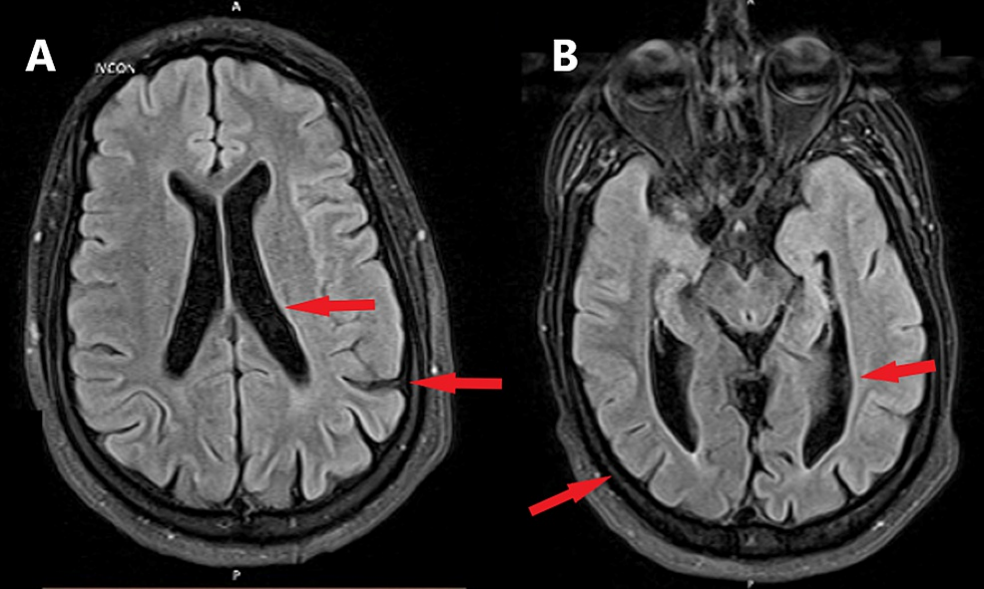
Axial brain MRI showing ventricular enlargement and diffuse cerebral volume loss most prominent in the bilateral parietal regions visible in both images (A,B) MRI - magnetic resonance imaging
